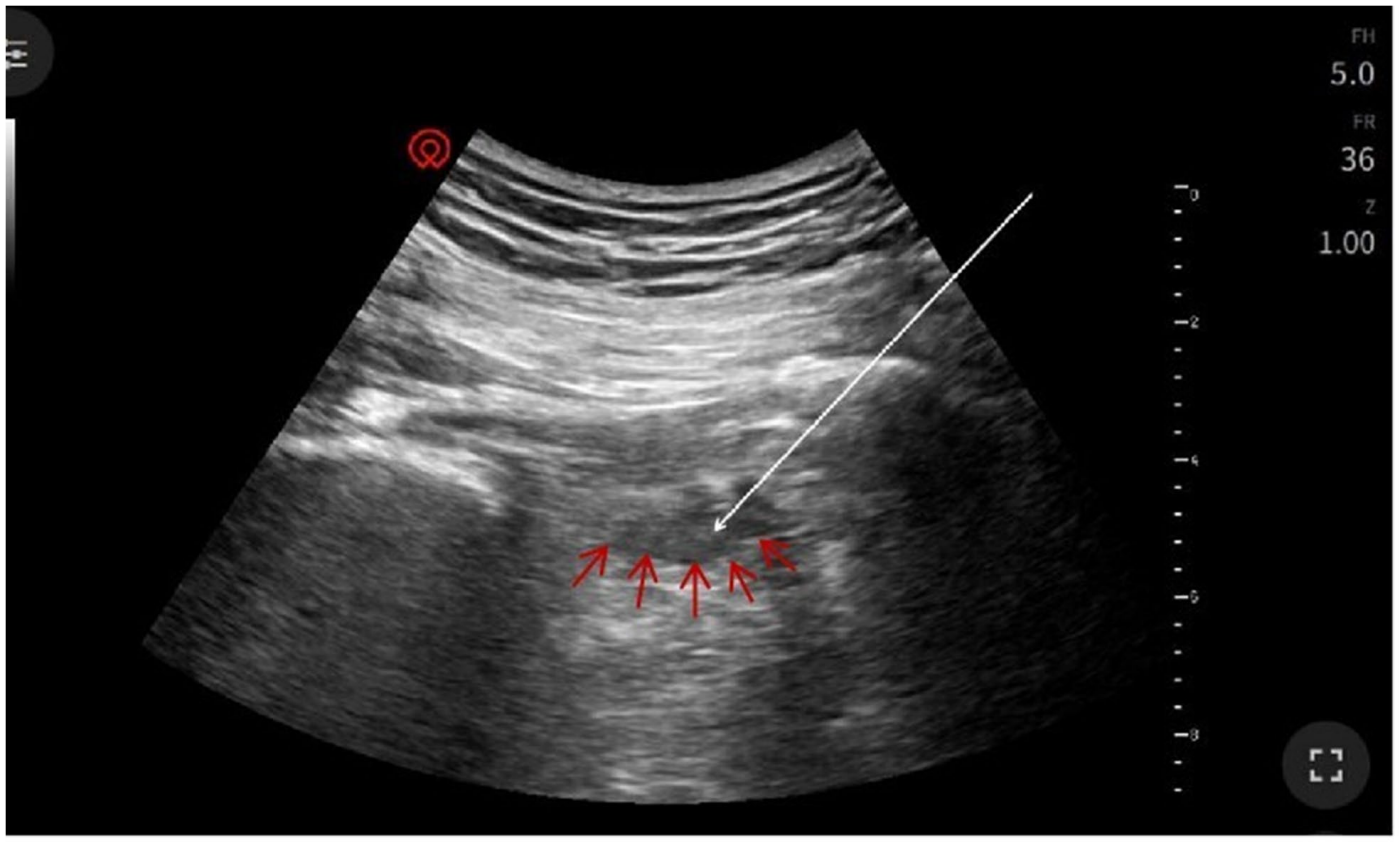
Ultrasound imaging of piriformis muscle: Left piriformis muscle (red arrow) demonstrating surrounding inflammatory signal with enhancement. Needle insertion site (white arrow) indicated at the tip of the arrow.
